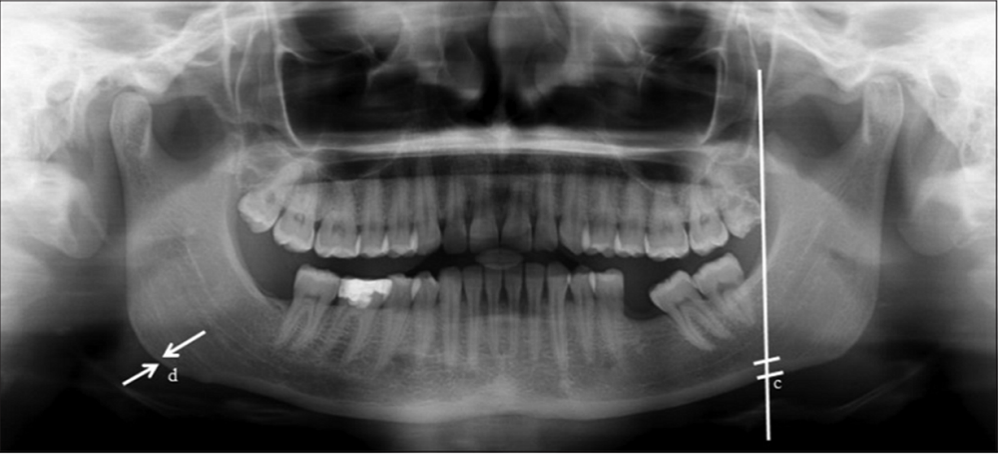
Radiographic representation of the determination of the antigonial index.
